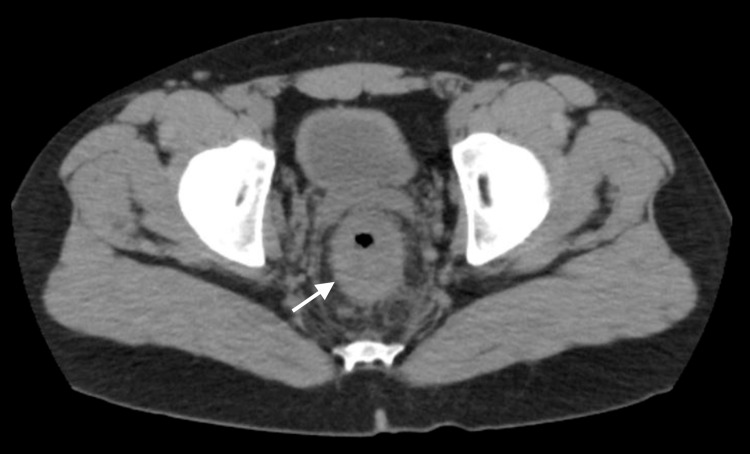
CT colonography with contrast shows significant thickening of the rectal wall, suggestive of rectal carcinoma.
